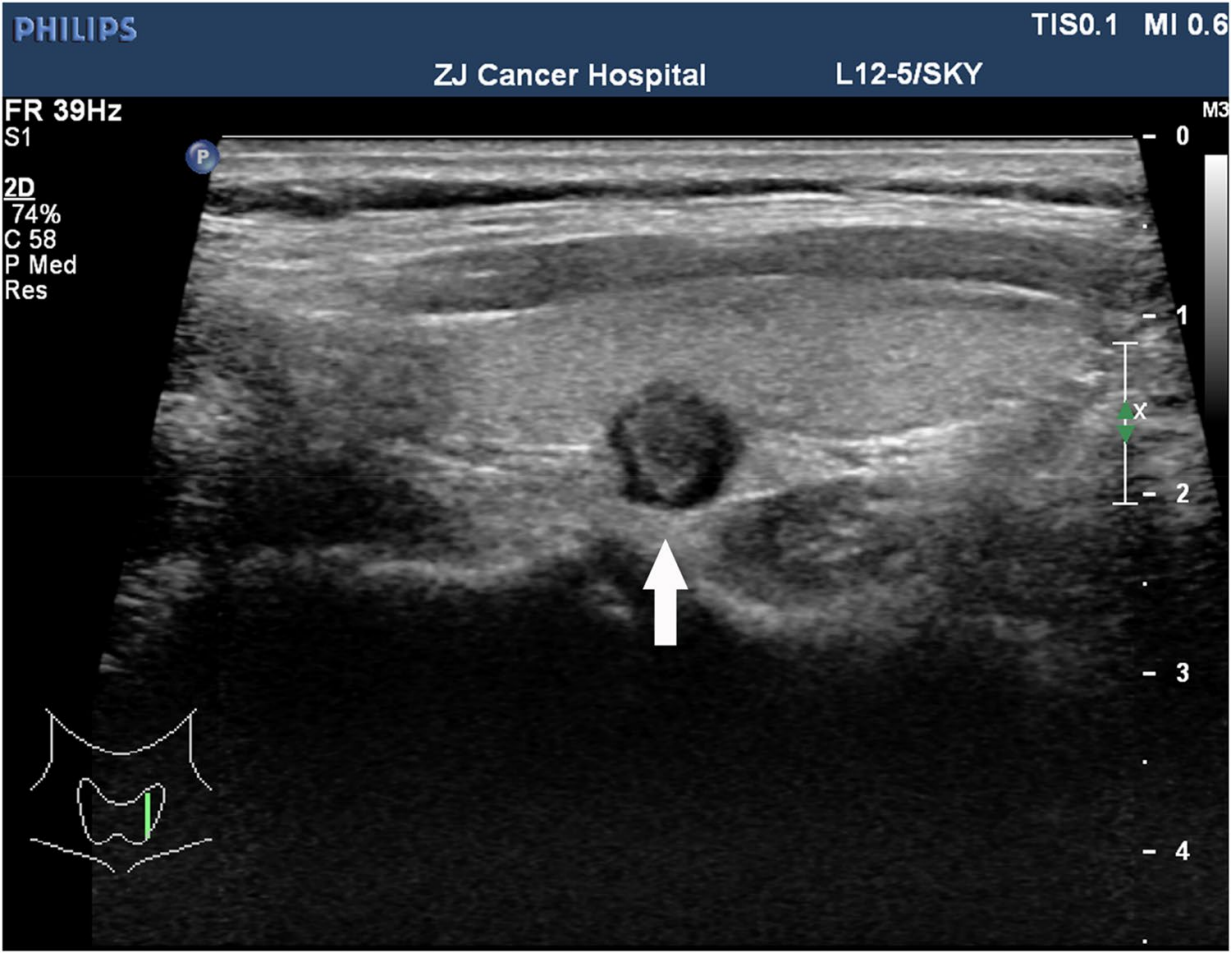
An ultrasound image of a 51-year-old female patient with papillary thyroid carcinoma.
